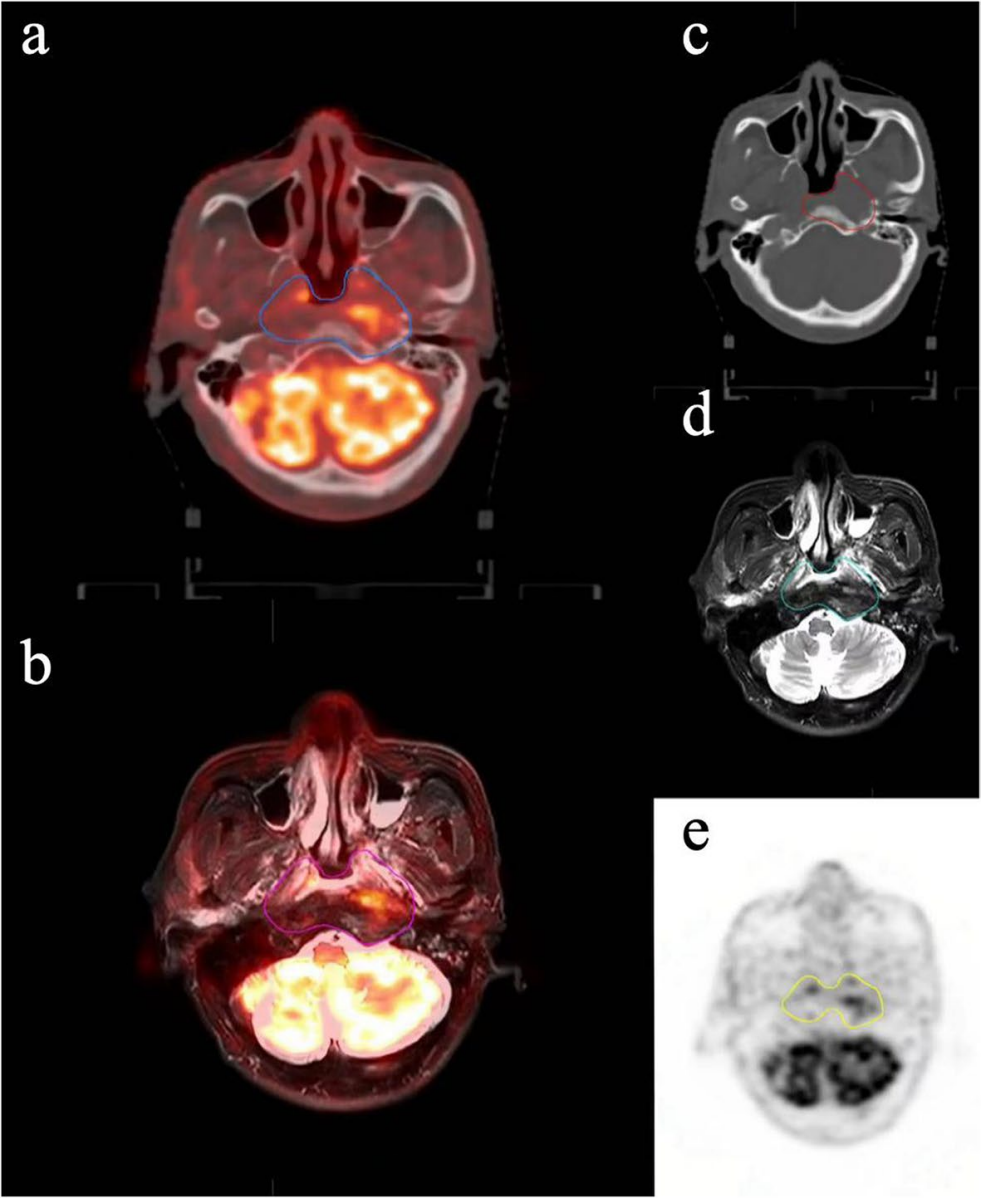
Sixty nine-year-old female with nasopharynx cancers. a The blue line represents gross tumor volume (GTV)-PET/CT. b The pink line represents GTV-PET/MRI. c The red line represents GTV-CT. d The green line represents GTV-MRI. e The yellow line represents GTV-PET
